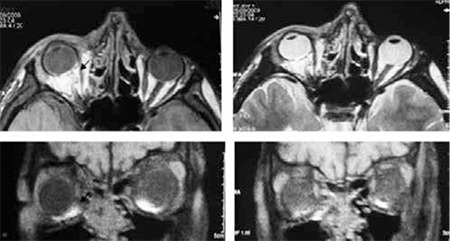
Case 2, MRI revealed enlarged right medial rectus due to hematoma.The medial rectus was paretic due to contusional trauma
